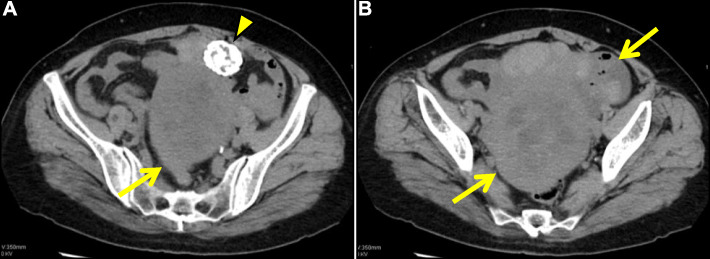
Non-contrast-enhanced abdominal/pelvic computed tomography. a and b: Axial images show bilateral inhomogeneous and well-circumscribed ovarian masses (arrows). Note the huge soft tissue mass with a central low attenuation area in the pouch of Douglas, which is bordered ventrally by a calcified uterine fibroid (arrowhead)
